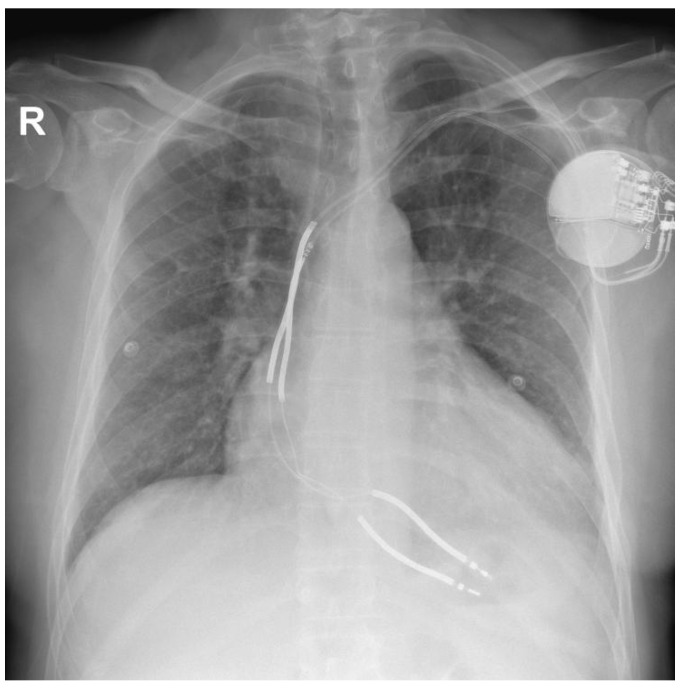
Chest X-ray at the time of admission at the hospital.
